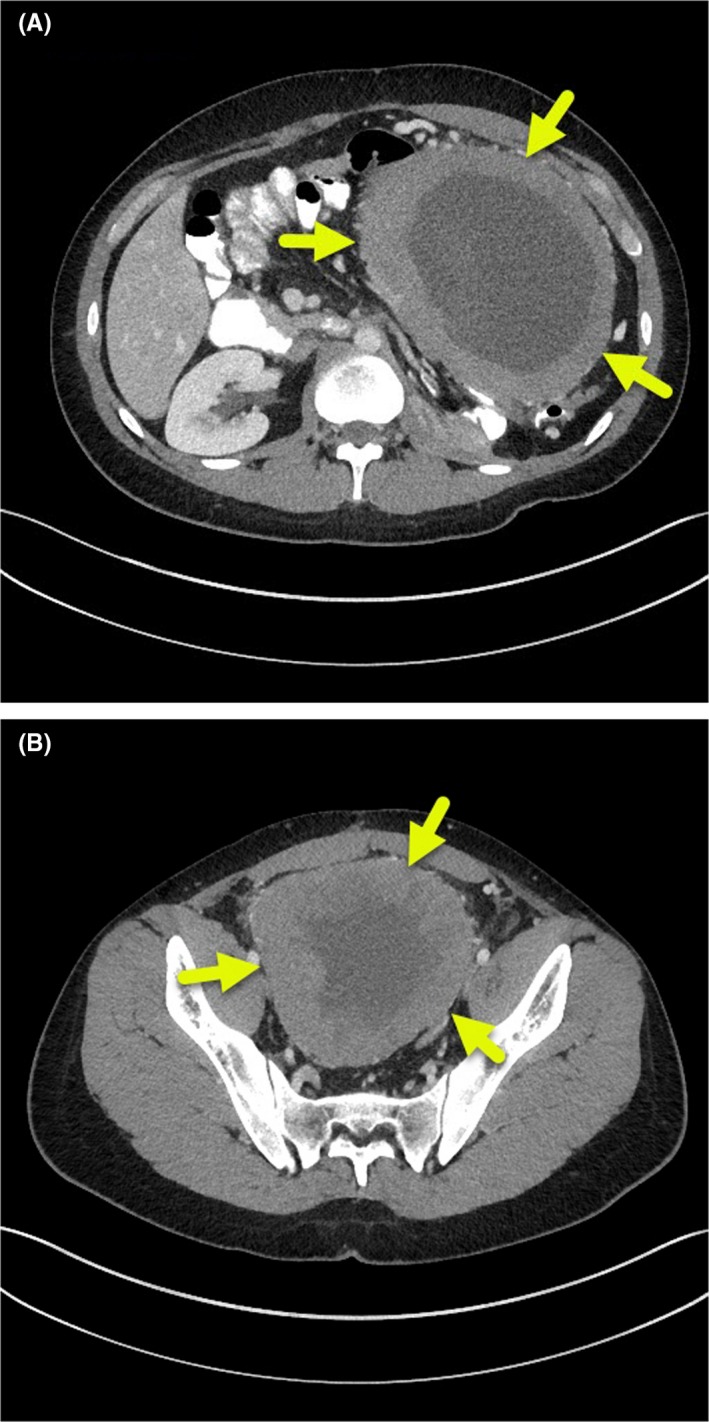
Representative image from an abdominal CT scan on 06/24/2013 showed a large, recurrent, partially necrotic abdominal pelvic mass with a thick rim (arrows)
